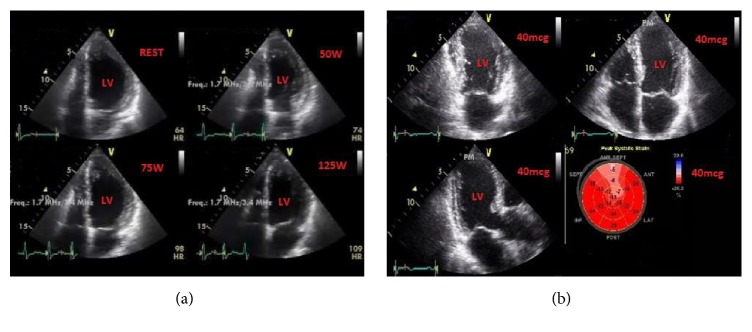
Provocative testing in ischemic heart disease. (a) Exercise stress echocardiography in a patient with normal coronary arteries. Persistent normal wall motion after exercise (125 W). (b) Dobutamine stress echocardiography in a patient with ischemic heart disease. Anteroseptal hypokinesia at peak dose (40 mcg) is shown both by wall motion abnormalities (top panels) and strain analysis (bottom right panel, lighter segments in the “bull's-eye” representation of peak systolic strain). LV = left ventricle.
